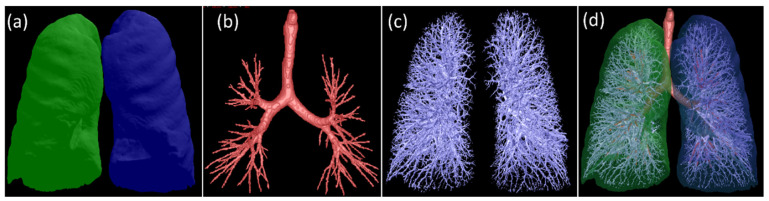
Example visualizations from a representative CT scan, including the segmented lung volumes (a), extracted airway tree (b), pulmonary vessels (c), and a combined rendering of all components (d).
